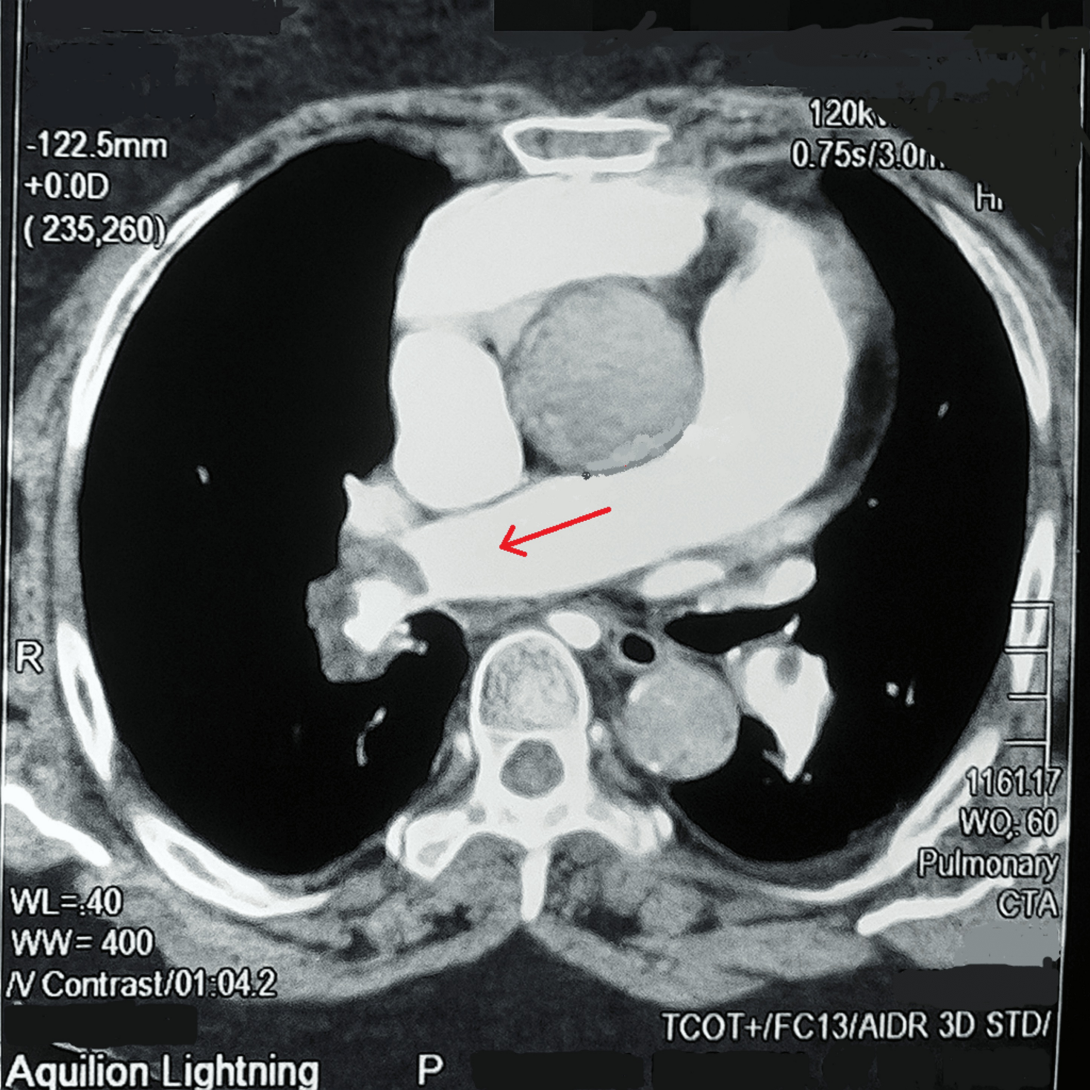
Computed tomography pulmonary angiogram (CTPA) demonstrating a filling defect in the right main pulmonary artery, partially occluding the vessel
